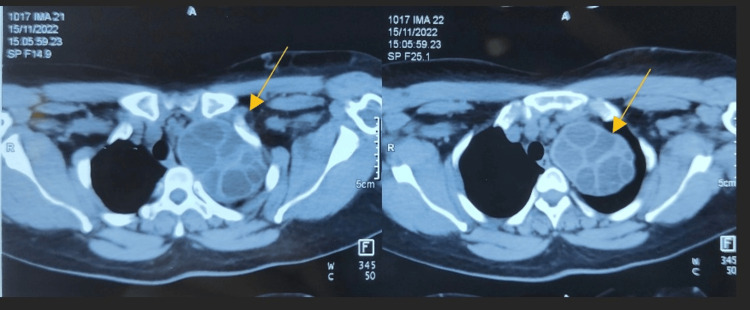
A CT scan of the thorax (cross-section) showing the multiloculated cystic lesion (pointed with the yellow arrow) extending through the thoracic inlet.
